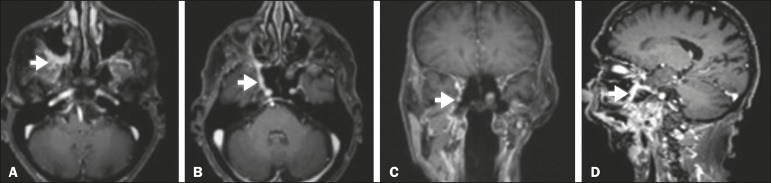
Gadolinium contrast-enhanced T1-weighted MRI scans of a 75-year-old man
